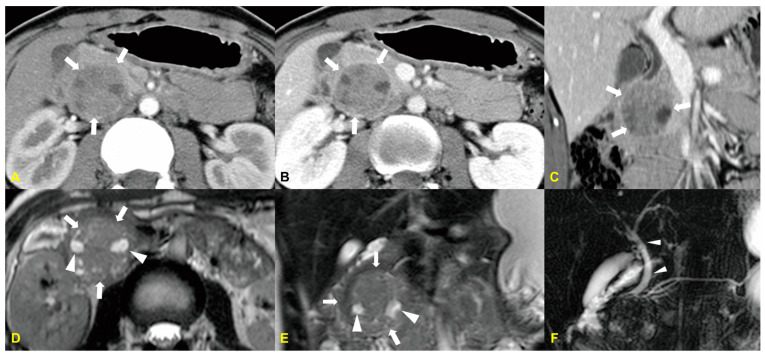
An 18-year-old woman with pancreatoblastoma. The contrast-enhanced arterial (A), delayed (B) phase, and coronal (C) CT images reveal a well-defined mass (arrows) with cystic and enhancing solid components in the head of the pancreas. The T2-weighted MR axial (D) and coronal (E) MR images show a heterogeneous mass (arrows) with internal high-signal-intensity foci (arrowheads), indicating cystic components. The 3D MR cholangiopancreatography image (F) reveals that the mass caused mild dilatation of the extrahepatic bile duct (arrowheads).
